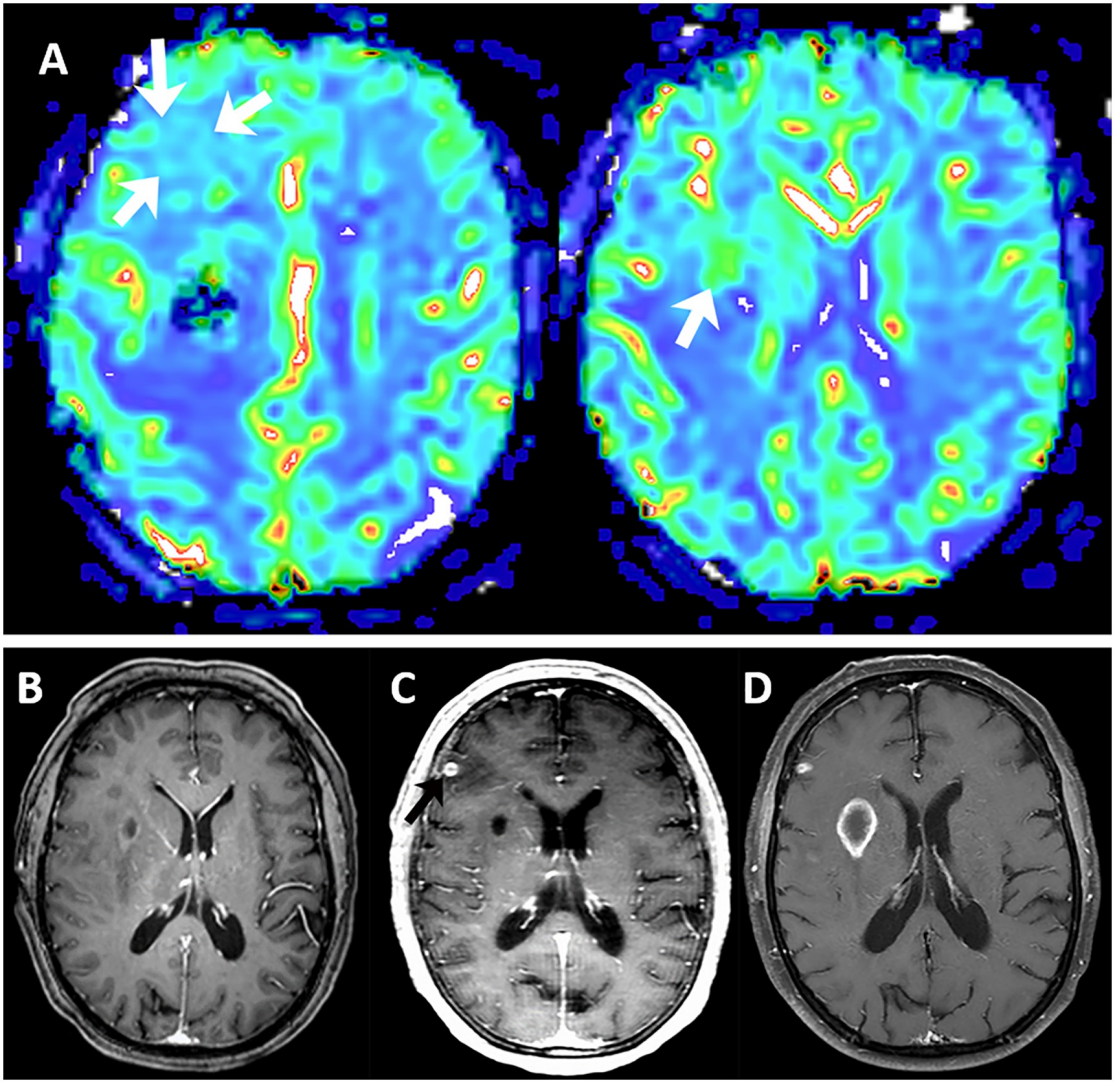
Patient with GBM and a near total resection. A). rCBV maps demonstrate focuses of elevated perfusion diffusely in the right frontal lobe and in the right basal ganglia (arrows). B) post-op T1 image with gadolinium demonstrates a cystic lesion with minimal enhancement in the right basal ganglia. C) 100 days post-operative MRI demonstrated tumor progression with development of a small nodule of cortical enhancement in the right frontal lobe (arrow). D) The next follow-up MRI demonstrates progression of the abnormalities with development of enhancement associated with the basal ganglia cystic lesion.
